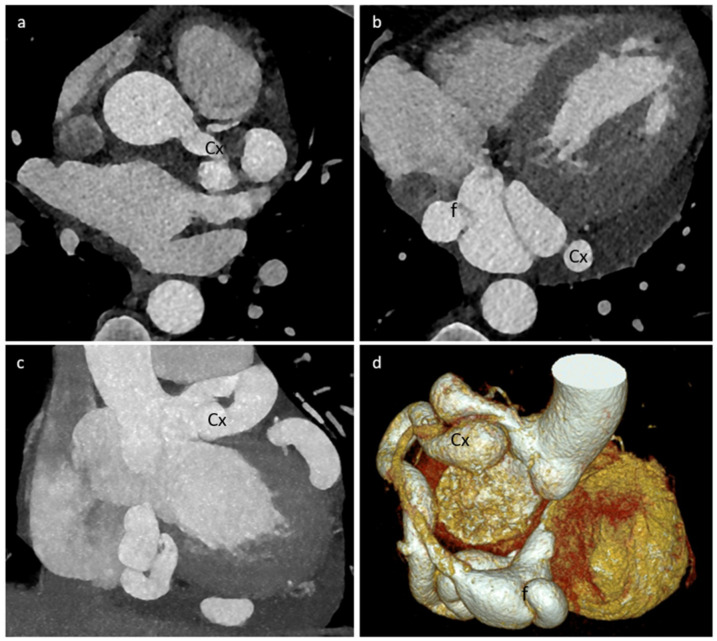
Circumflex artery (Cx) to coronary sinus fistula (f) at axial (a,b), maximum intensity projection (c) and volume-rendering (d) computed tomography images.
